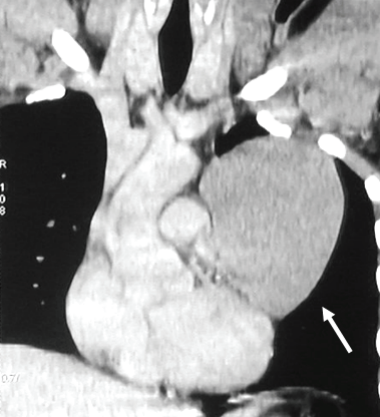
CT scan showing cystic mass. CT scans showing a well circumscribed cystic mass (10 × 10 cm) adjacent to the left pulmonary artery (arrow).
